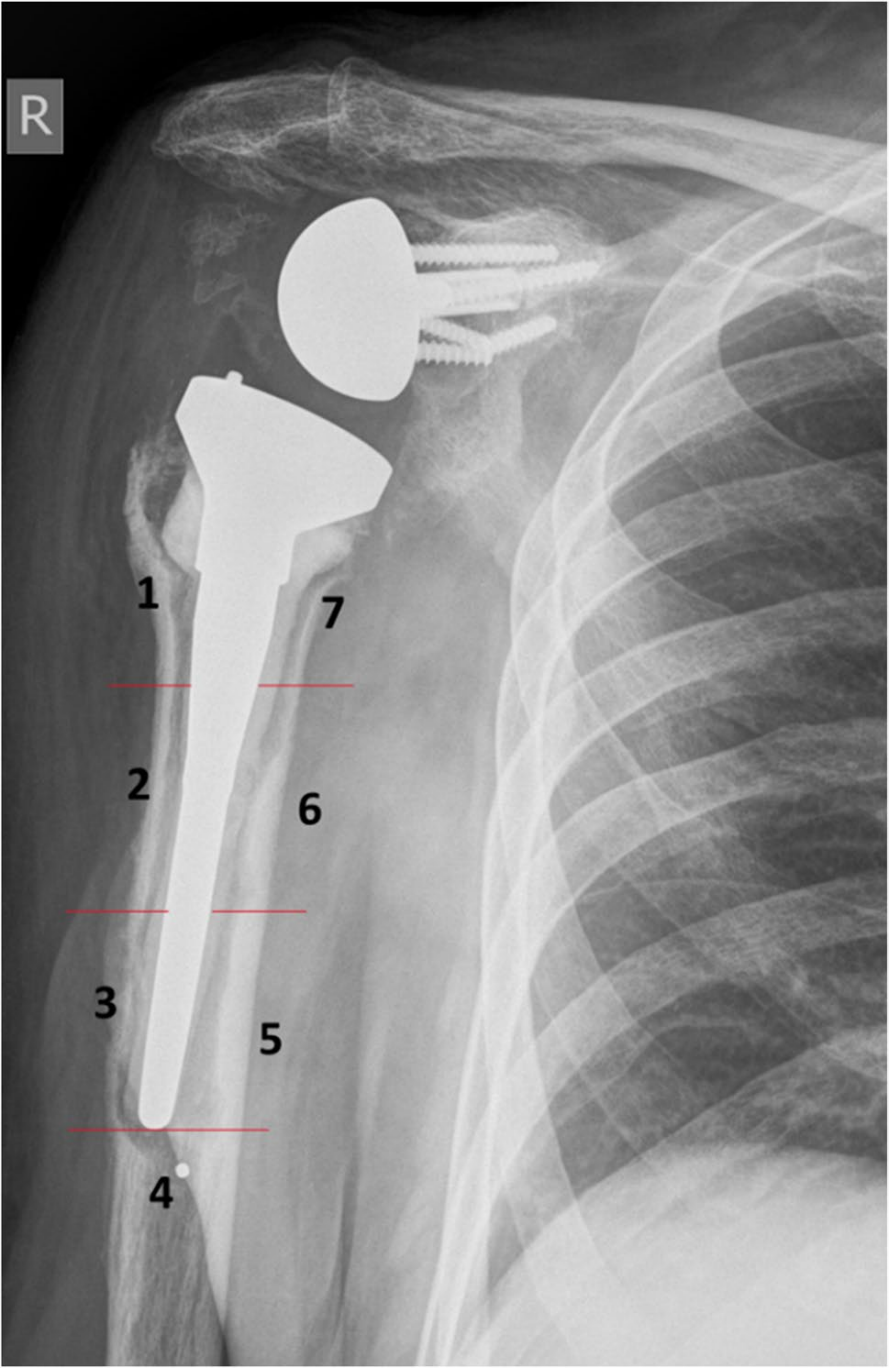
Antero-posterior radiograph of a right shoulder with RSA. The humeral zones according to the classification described by Boileau et al. are labeled 1–7 [5]. In the presented case, RLL are present in all zones
